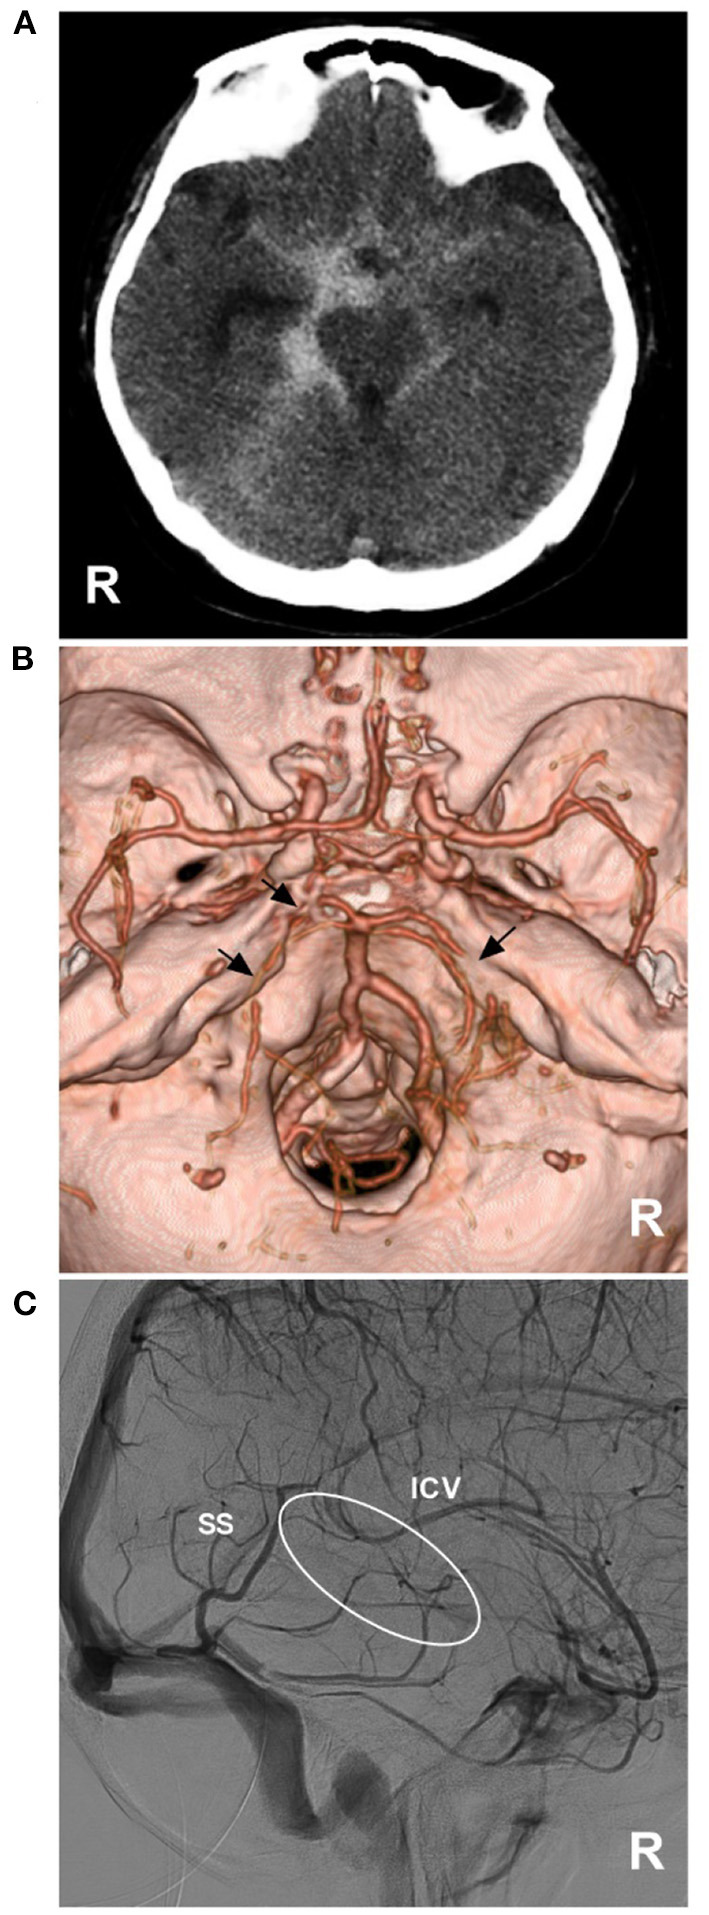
PNSAH with BVR absence. (A) CT showing a PNSAH that extended to the posterior part of the anterior interhemispheric fissure and the basal part of the Sylvian fissure. (B) CTA showing the multiple vasospasms (arrows) of the bilateral posterior cerebral arteries, without positive findings of bleeding. (C) DSA showing the absence of the right BVR (circle). BVR, Basal vein of Rosenthal; CT, computed tomography; CTA, computed tomography angiography; DSA, digital subtraction angiography; ICV, internal cerebral vein; PNSAH, perimesencephalic non-aneurysmal subarachnoid hemorrhage; R, right; SS, straight sinus.
